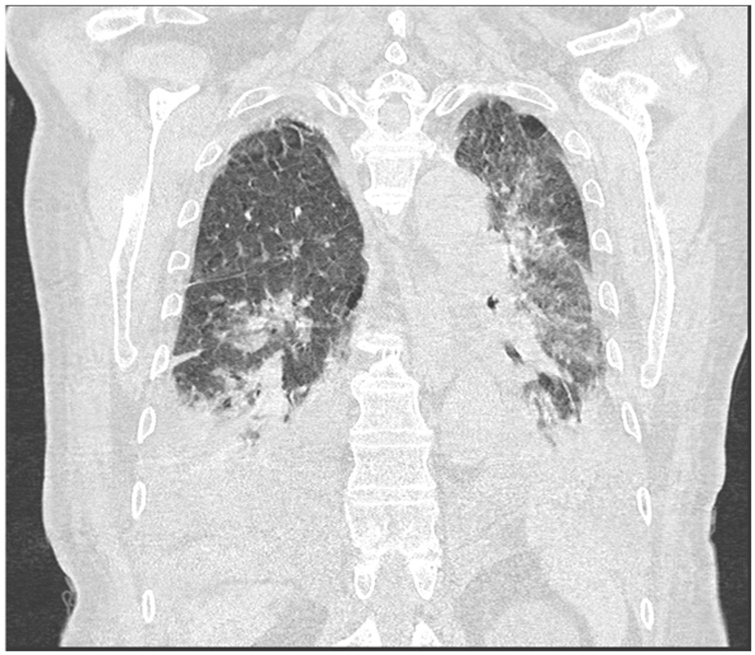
High-resolution computed tomography scan of lung showing extensive bilateral interstitial pneumonitis.
